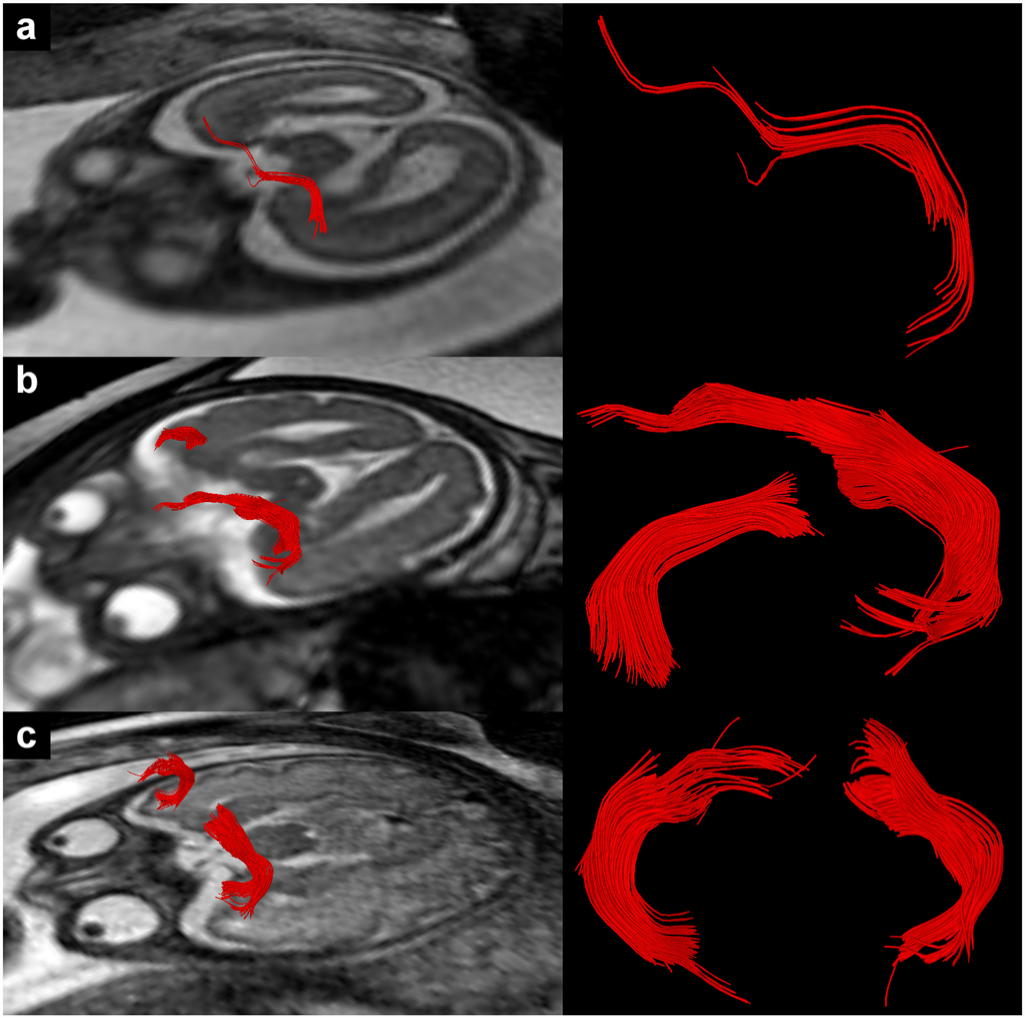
In utero tractography of the UF in subjects 3 (a), 16 (b) and 24 (c) (22, 28 and 34 GW respectively). Fiber tracts are projected on axial T2-weighted images.
